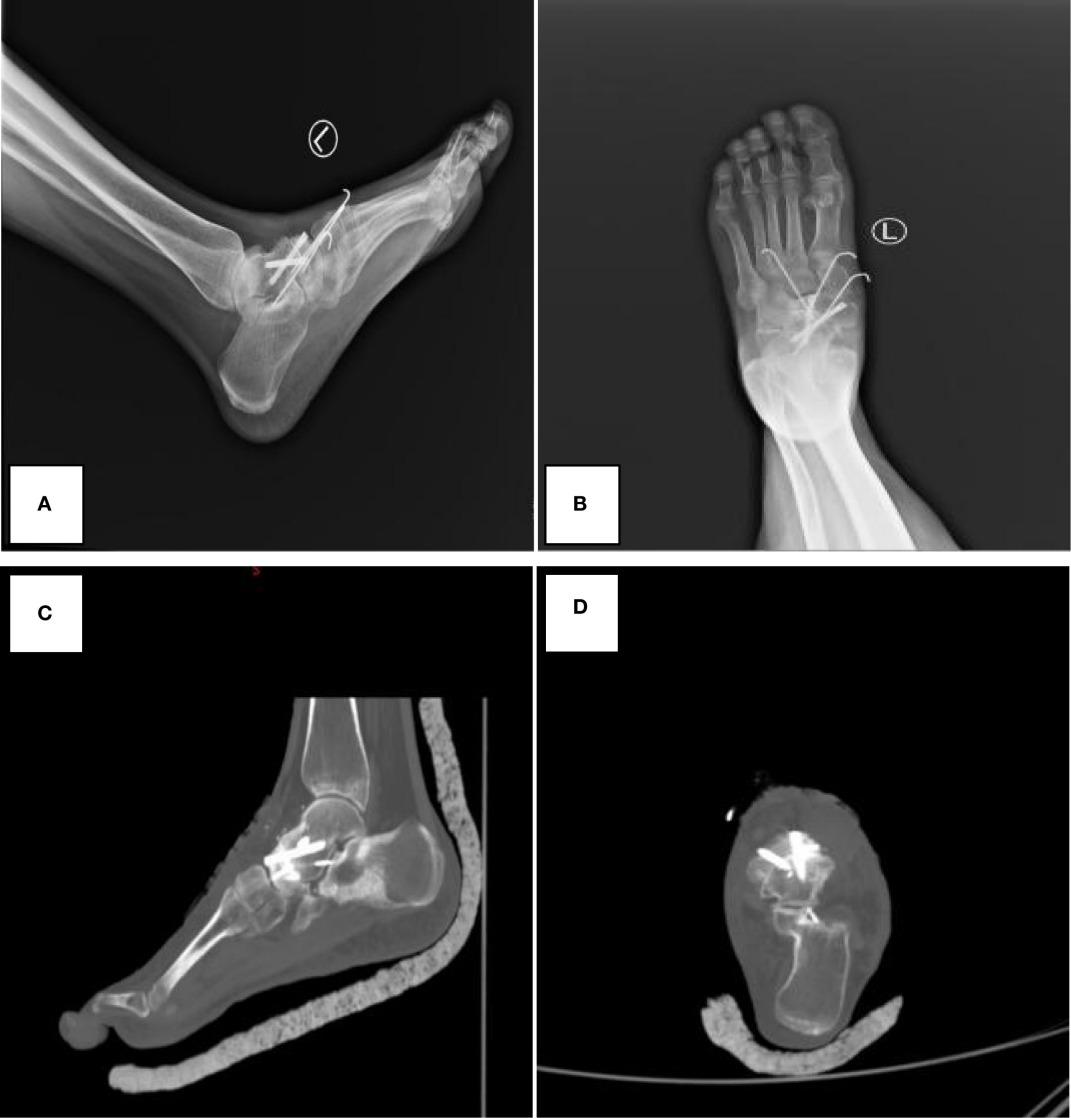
Postoperative imaging demonstrating successful talonavicular joint fusion with hardware *in situ*. (A, B) Postoperative X-ray images. (C, D) Postoperative CT images. The images demonstrate good alignment of the talonavicular joint and internal fixation.
